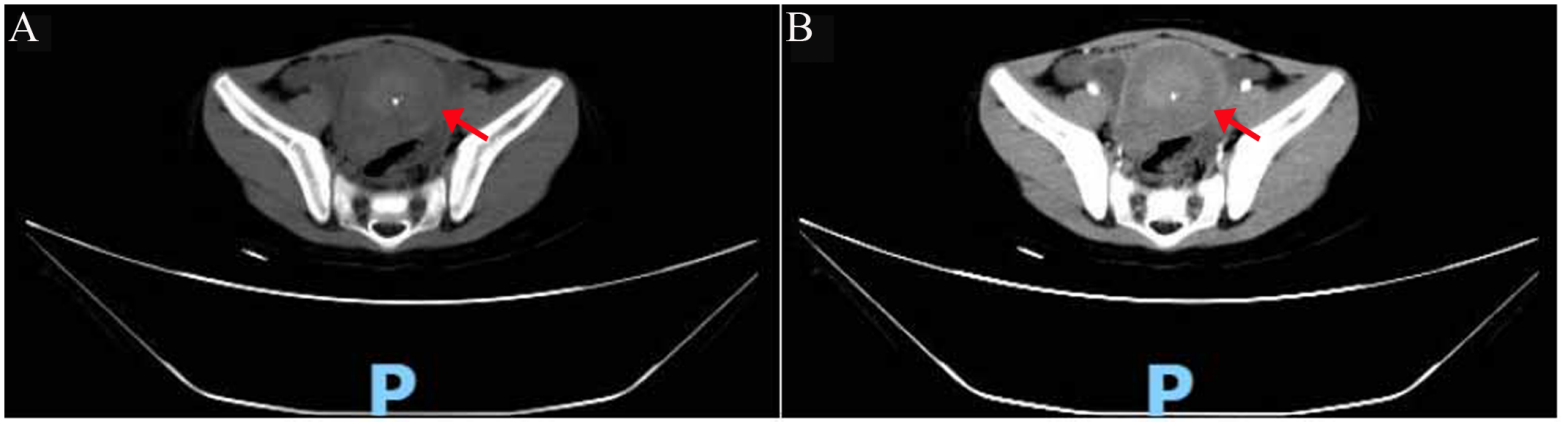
CT scans. (A) Plain CT: mixed-density mass in the abdominopelvic cavity (red arrow). (B) Contrast-enhanced CT: heterogeneous and peripheral enhancement (red arrow). CT, computed tomography.
